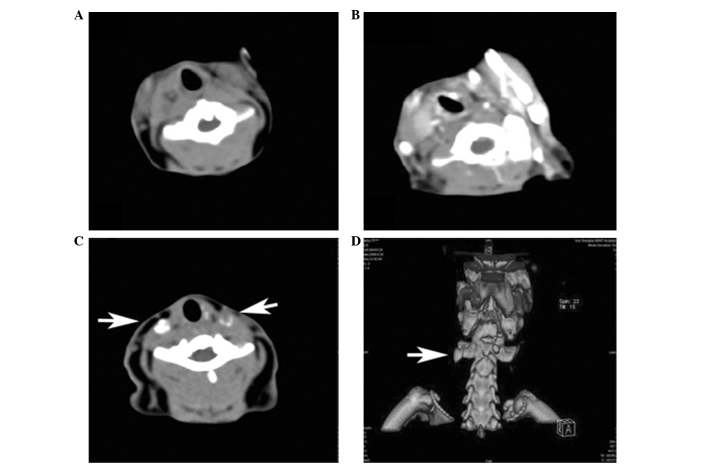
Imaging findings of cervical lymph nodes. (A) On plain CT images, cervical lymph nodes were not clearly identified. (B) Cervical lymph nodes were also not clearly identified in the venous enhancement. (C) By CT lymphography, the contrast agent filling defected appeared on the metastasis nodes. (arrow) (D) The oval-shaped lymph nodes (arrow) with greater diameters than those of the lymphatic vessels were easily identified on three dimensional images. CT, computed tomography.
